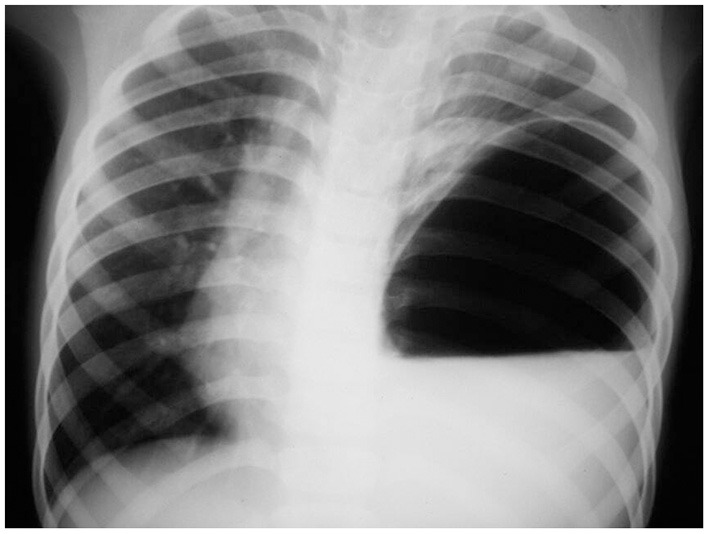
The plain chest x-ray shows an air-fluid level on the left side of the chest with an absent gastric shadow, initially interpreted as hydropneumothorax.
